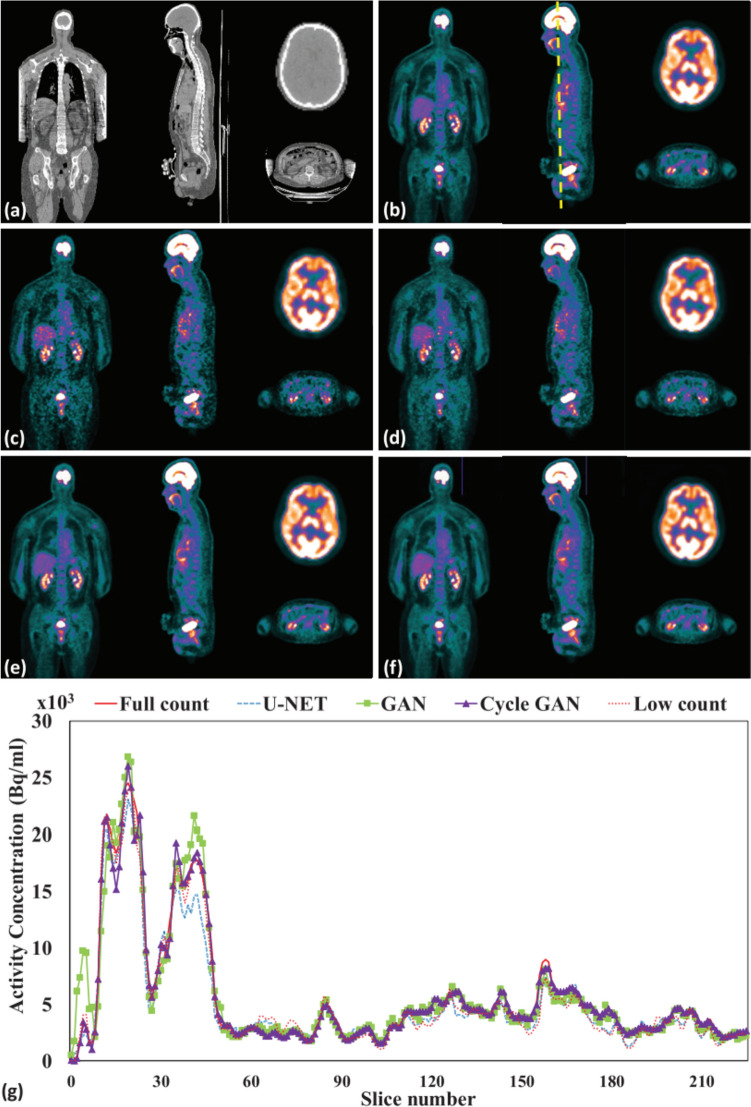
Examples of the denoised whole body.^18^F-FDG PET images by supervised learning approaches. Sample images showing (a) CT, (b) full-count PET, (c) low-count PET, and denoised PET images corresponding to the (d) U-Net, (e) GAN, and (f) CycleGAN. (g) Line profiles in sagittal section. © 2019 IOP Publishing. Reprinted with permission from Lei et al. [127]
